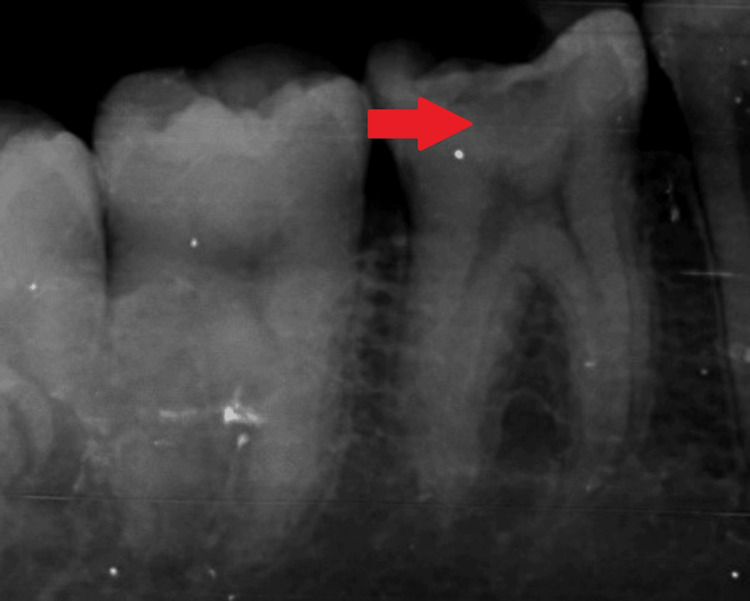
Preoperative intraoral periapical radiograph The arrow shows radiolucency involving enamel, dentin, and approaching pulp.
